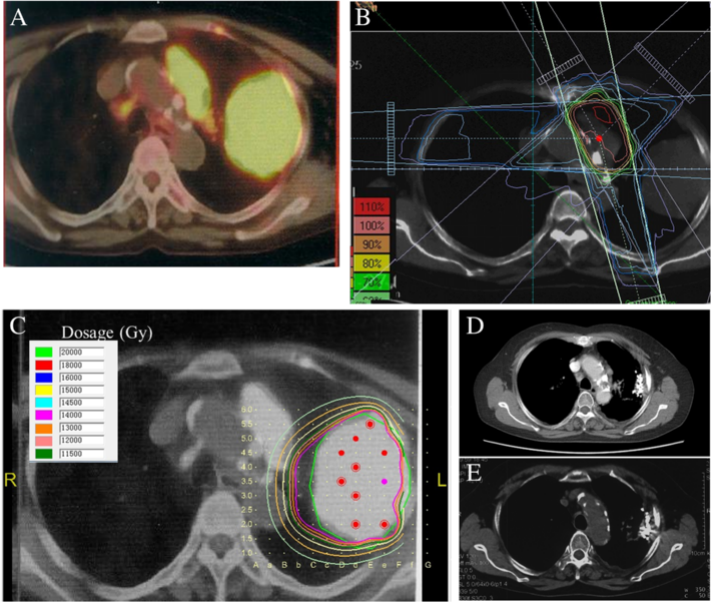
PET-CT image of a massive squamous cell carcinoma (7 × 5 cm^2^) in the upper left lung with metastasis in the superior border of the pericardium and mediastinum (3 × 4 cm^2^) in a 65-year-old woman (No. 13) (a). The metastasis region was treated with EBRT at a dose of 30 Gy (b) and the massive lung cancer region was implanted with iodine-125 seeds (red and purple dots) for brachytherapy (c) guided by CT. Thoracic CT image was tumor-free at 1-year follow-up (d), and showed no recurrence of cancer at 3-year follow-up (e)
